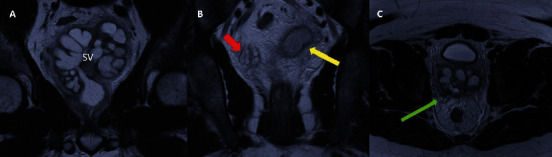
(A–C) A 25-year-old male presents with abdominal pain, dysuria, and constipation. (A) Coronal T2 MR image demonstrates a T2 hyperintense mass representing a dilated left seminal vesicle (SV). (B) Coronal T2 demonstrates a normal appearing right seminal vesicle (red arrow). The partially visualized left seminal vesicle is dilated (yellow arrow). (C) Axial T2 shows a normal appearing right ejaculatory duct (green arrow). The left ejaculatory duct is not visualized and demonstrates atresia of the ejaculatory duct. There is T2 hypointense signal layering within the dilated left seminal vesicle likely representing debris.
